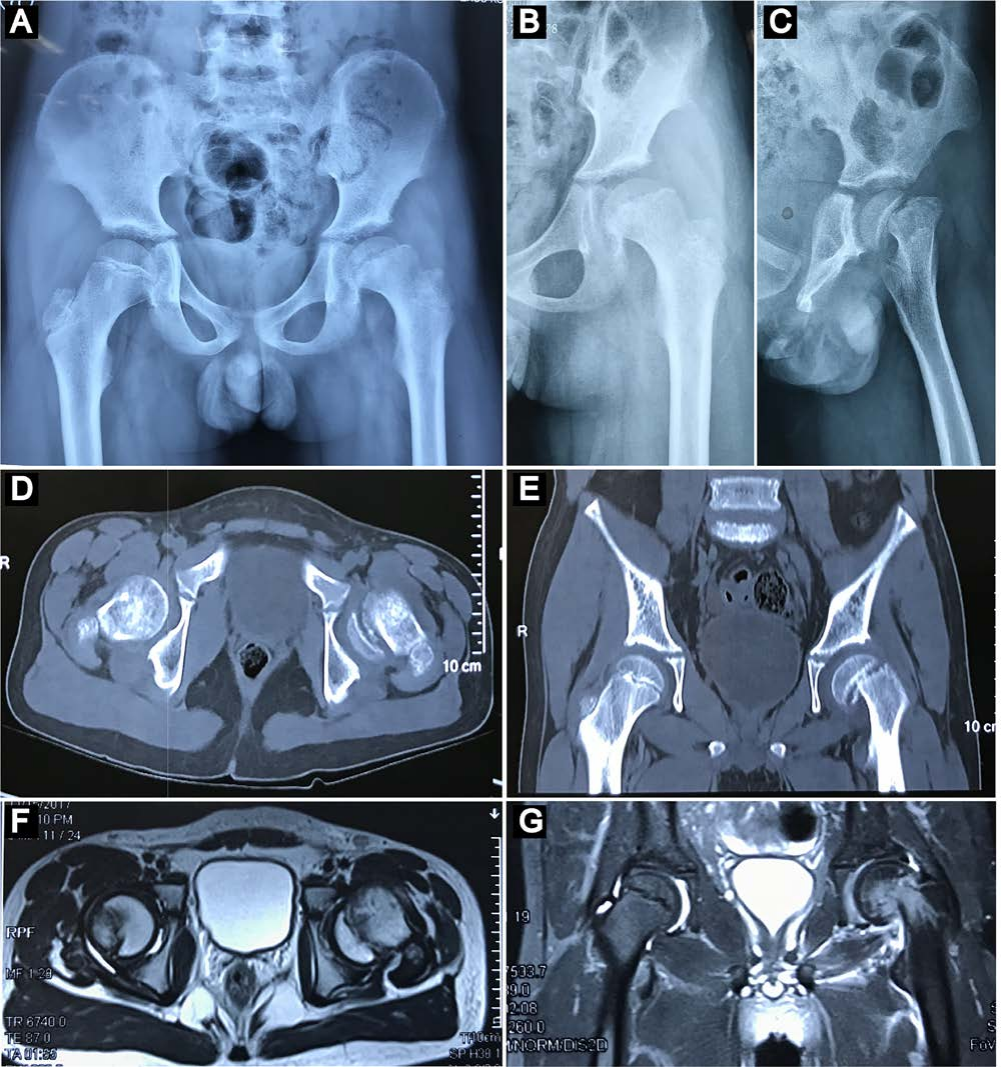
Imaging data at admission. (A) Antero-posterior pelvic radiograph. (B) Antero-posterior radiograph of the left hip. (C) Lateral radiograph of the left hip. (D) Axial plane of CT scan. (E) Coronal plane of CT scan. (F) Axial plane of MRI. (G) Coronal plane of MRI. MRI = magnetic resonance imaging.
